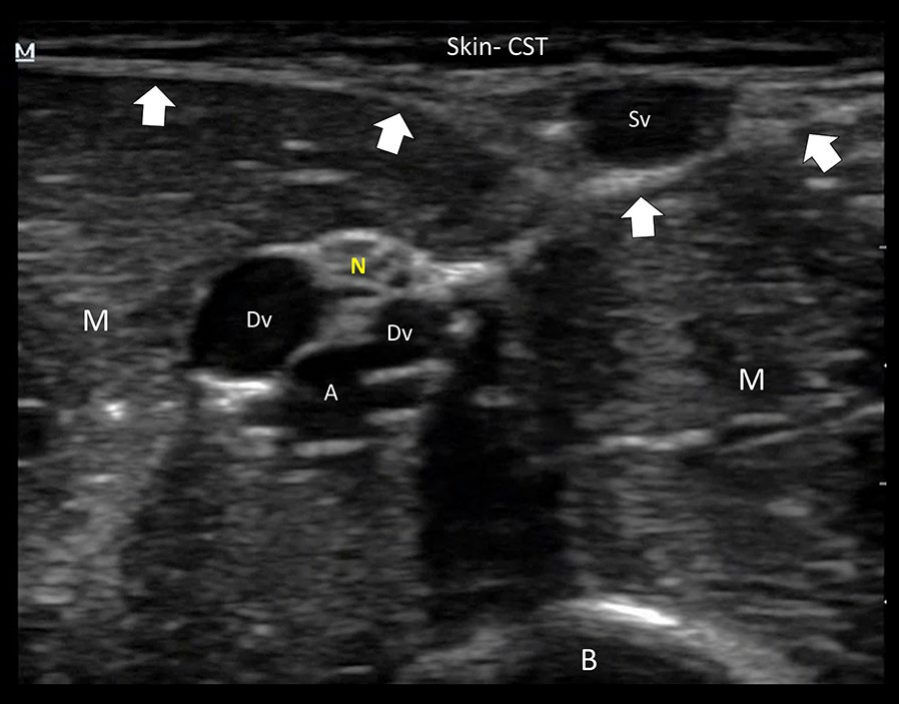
Ultrasound anatomy applied to cannulation of the peripheral veins of the upper limbs. Superficial veins (Sv) are found above fascia (delineated by arrows) in the cellular subcutaneous tissue (CST), and deep veins below fascia, or subfascial. As noted, deep veins are paired (brachial veins in this case), and are accompanied by an artery (A, brachial artery in this example) and a nerve (N, median nerve in this case) in the neurovascular bundle. The muscle (M) and the bone (B) are also found in the subfascial compartment. Superficial veins should be selected for cannulation
